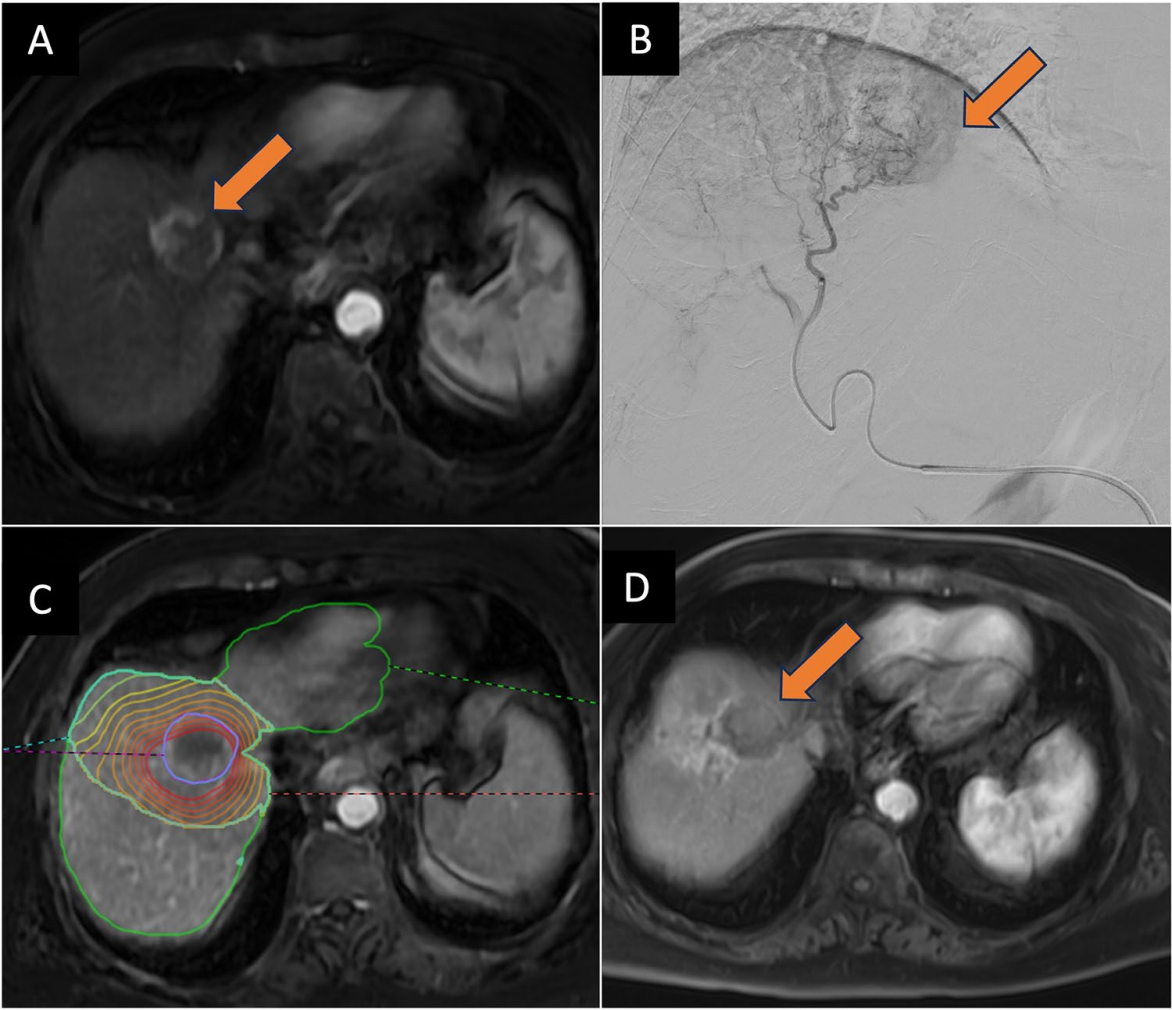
a Axial arterial phase magnetic resonance image (MRI) of the abdomen, demonstrating a segment 8 HCC (orange arrow) b Digital subtraction angiogram during planning angiography with the tip of the microcatheter positioned within the proximal segment 8 artery. Tumor enhancement is seen distal to the catheter as demonstrated by the orange arrow. c Post-Y90 delivery dose contour map generated using Simplicit90Y image-fusion and dosimetry planning software d Three-month follow-up axial arterial phase MRI of the treated tumor, demonstrating a complete response to radioembolization with adjacent post-radiation changes (Color figure online)
